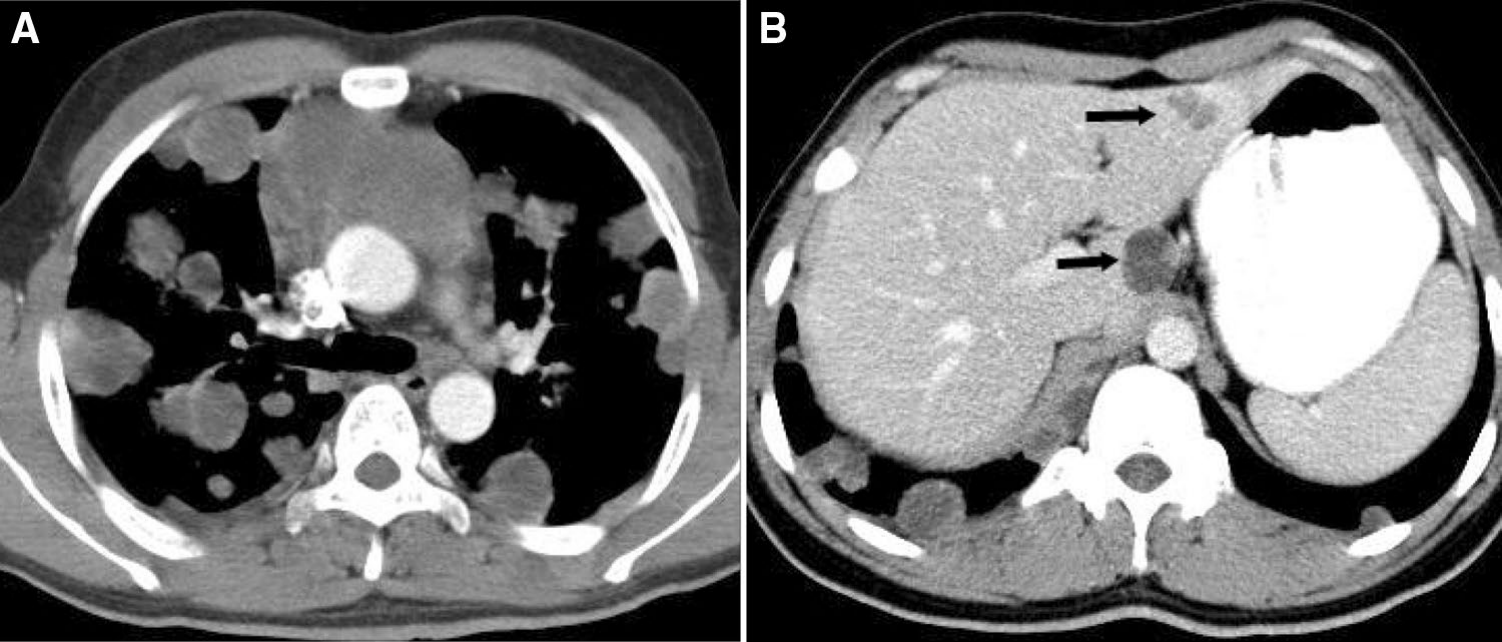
Thymic squamous carcinoma in a 27-year-old man withlung and liver metastasis. Contrast enhanced CT scan (Axial 5.0 mm) obtained at the level of the main bronchi (A) and the first porta hepatis (B) shows multiple metastasis in the bilateral lung and the left lobe of the liver (black arrow). This patient died 26.1 months since the first CT examination.
